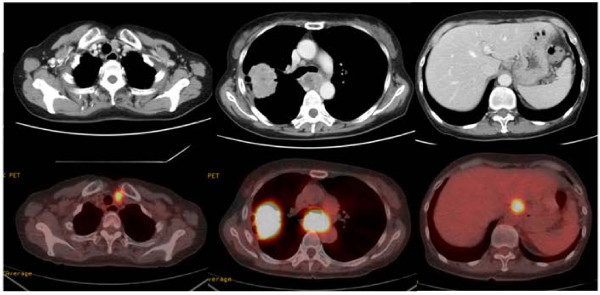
Chest computed tomography (CT) scan. The CT scan reveals the mass in the right upper lobe and enlarged lymph nodes of the upper mediastinum, around the upper thoracic esophagus and lesser curvature of the stomach. Positron emission tomography (PET)-CT reveals positive findings of the same lesions as the CT scan with no other positive lesion (maximum standardized uptake value: lung tumor 19.5, lymph nodes #2 L 9.3, #7 24.3, lesser curvature of the stomach 13.2).
